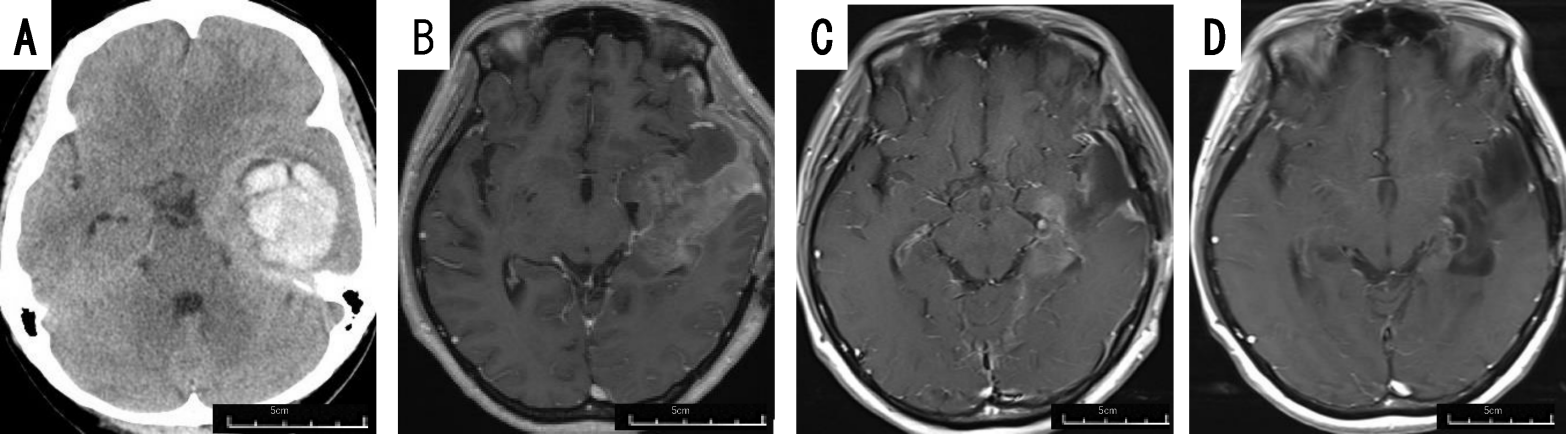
Patient 9 (40-45 year-old woman): GBM, *IDH*-wild, presenting with intratumoral hemorrhage. Preoperative computed tomography image (A) of patient 9 displaying intracerebral hematoma in the left temporal lobe, requiring evacuation. Gadolinium-enhanced brain MRI taken immediately before tumor removal (B), and 1 month (C) and 24 months (D) after tumor removal.
